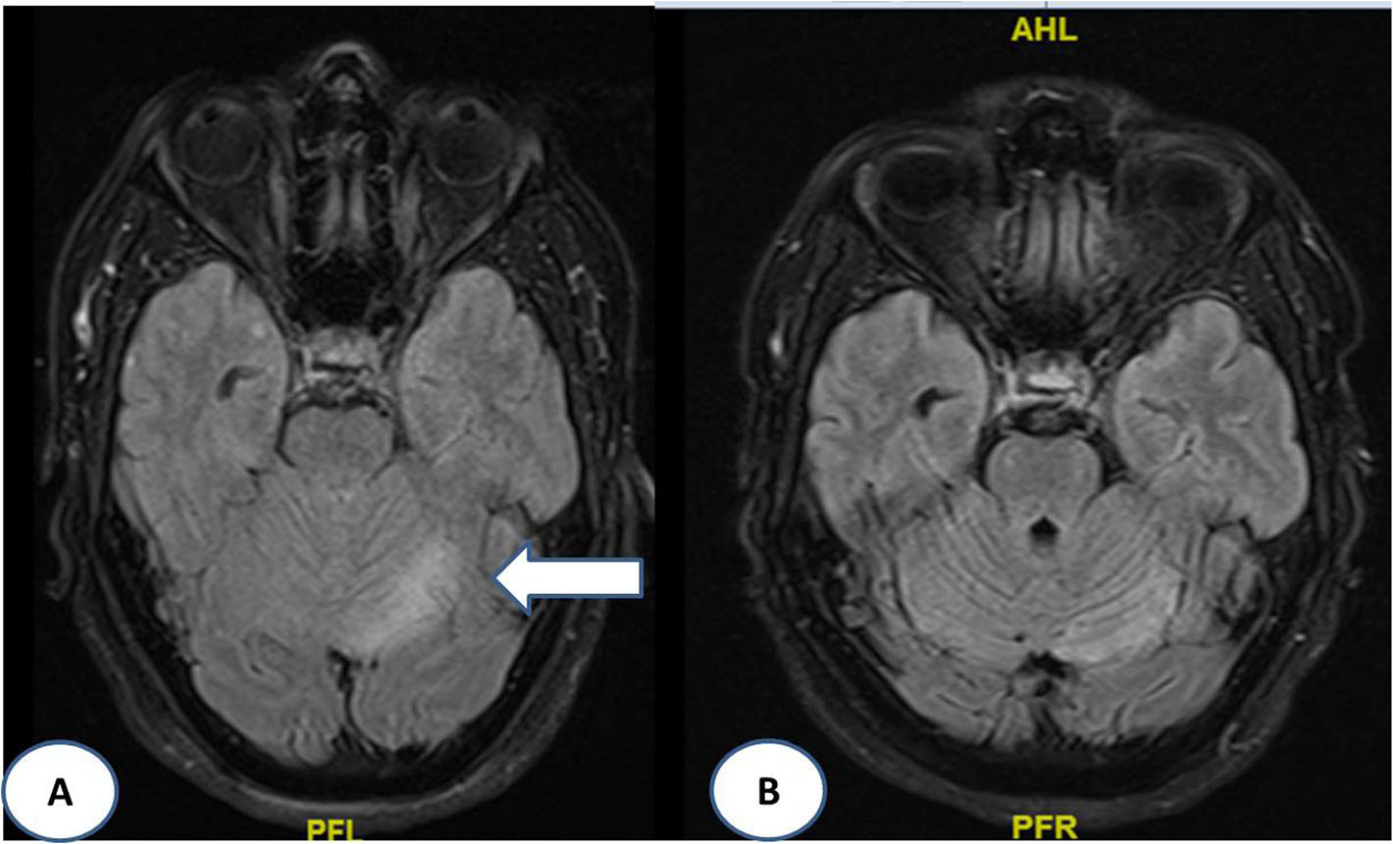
a MRI (axial), FLAIR sequence showing hyperintesity at left cerebellum. b MRI (axial), FLAIR sequence showing disappearance of hyperintesity
